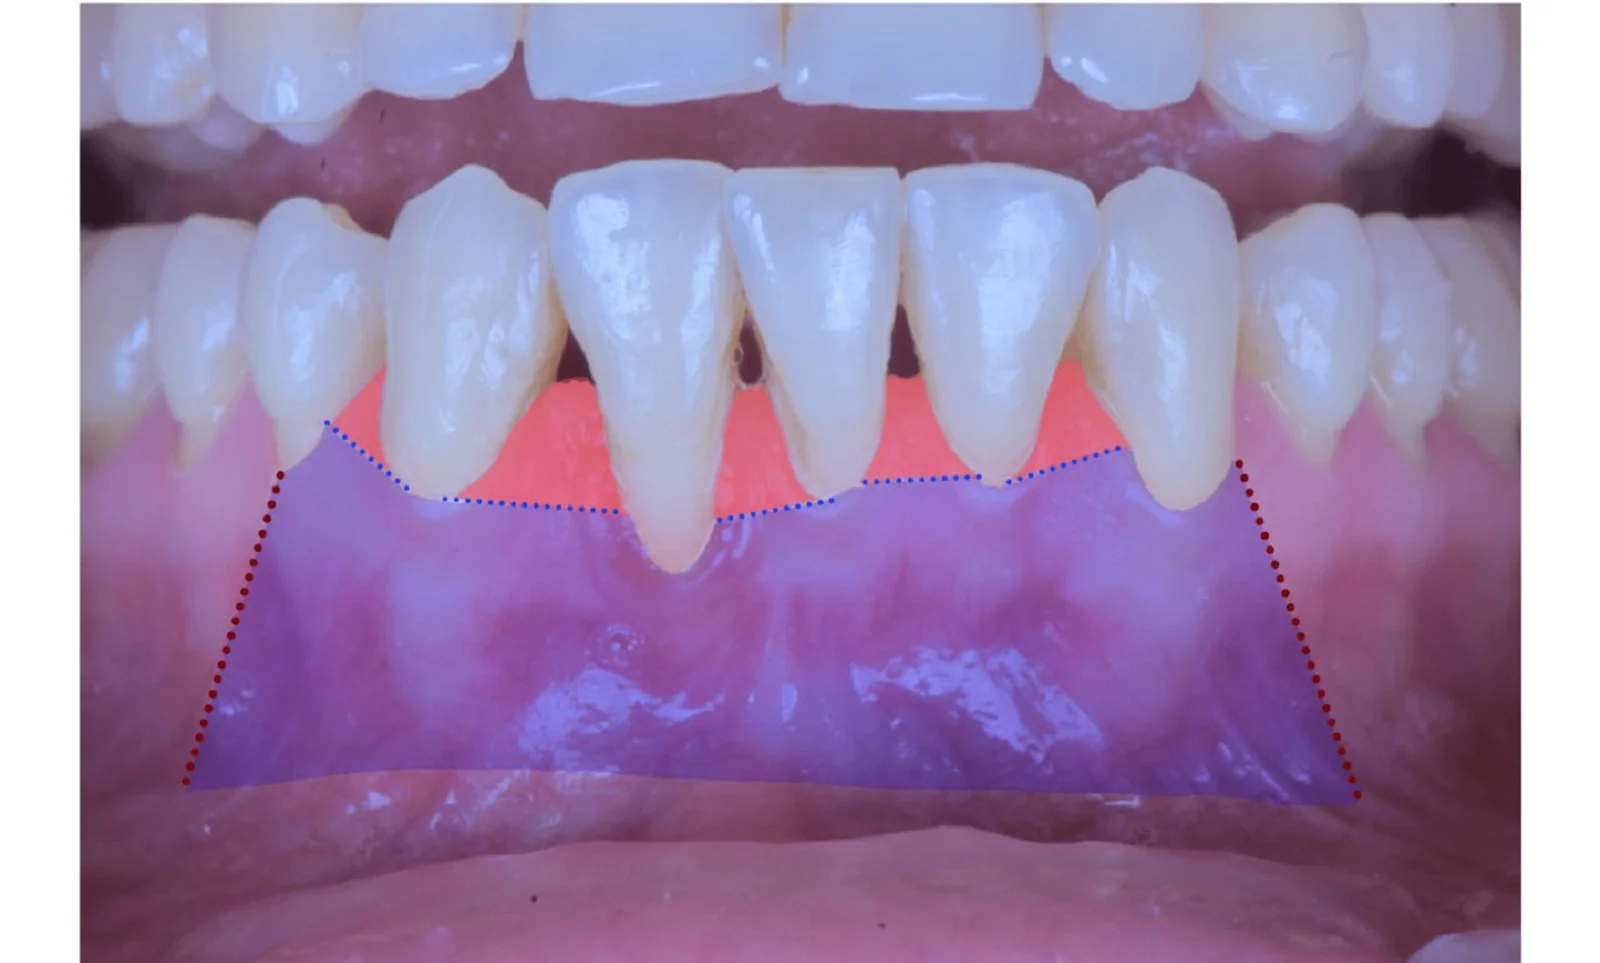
Surgical flap design based on CBCT evaluation. Preoperative design of the coronally advanced flap planned using the clinical examination and cone beam computed tomography (CBCT) findings. The incision outlines and flap extension were designed according to the areas of facial alveolar bone deficiency identified on CBCT to allow adequate flap mobilization, passive coronal advancement, and complete coverage of the connective tissue graft over the mandibular anterior gingival recessions.
